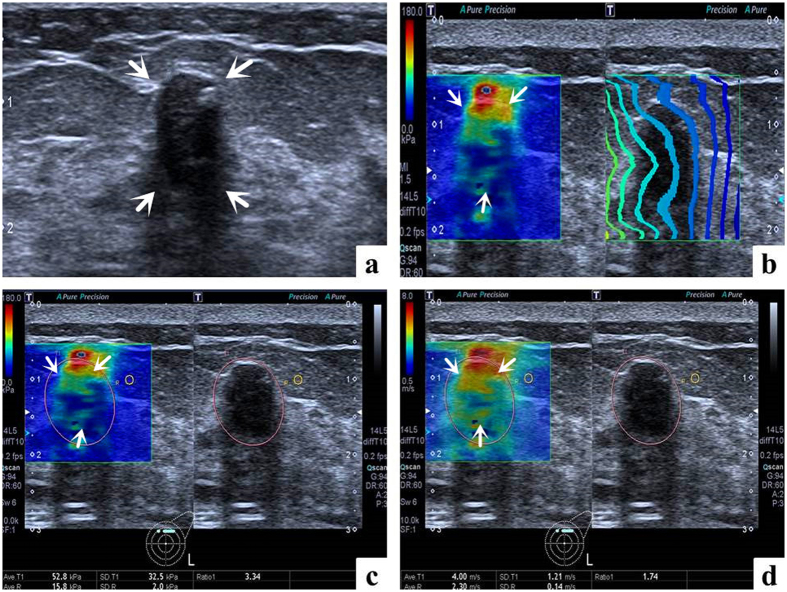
False positive case images in a 62-year-old woman with fibroadenomatous hyperplasia. (a) The lesion (arrows) is shown on B-mode ultrasound. (b) The lesion (arrows) shows irregularly distorted and chaotic contour lines on the shear wave propagation mode. (c) The Emean, E_SD_ and Eratio of the lesion (arrows) on elasticity mode are 52.8 kPa, 32.5 kPa and 3.34 respectively. (d) The Emean, E_SD_ and Eratio of the lesion (arrows) on shear wave speed mode are 4.00 m/s, 1.21 m/s and 1.74 respectively.
